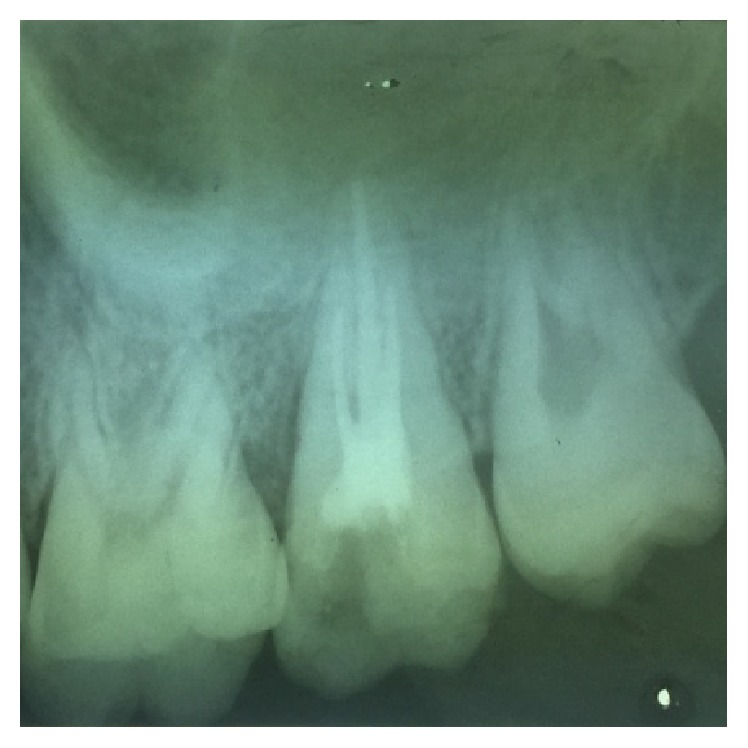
Postobturation radiograph with respect to 27.
